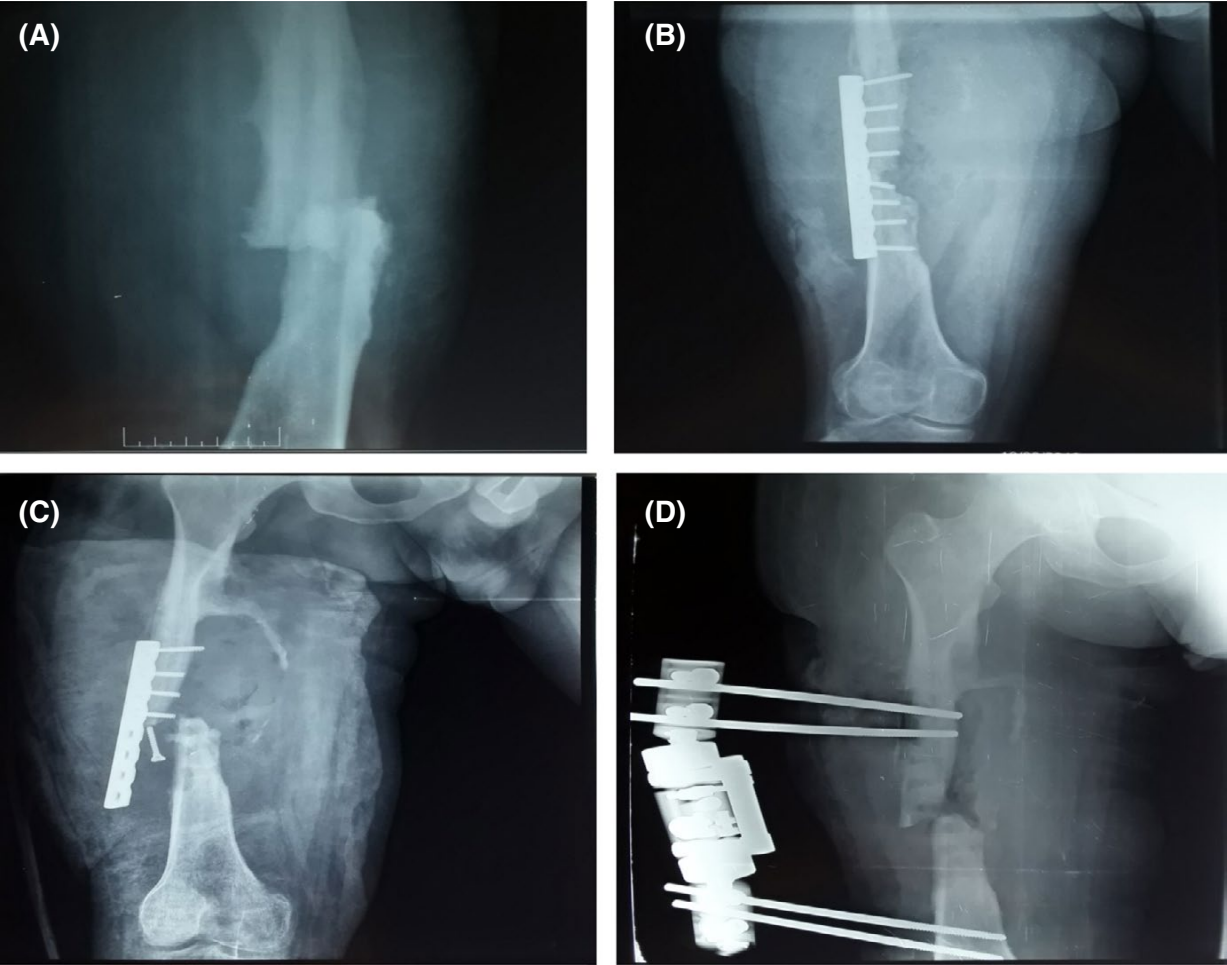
A, Anteroposterior (AP) radiograph demonstrating a comminuted femoral shaft fracture in a 37‐y‐old male patient with hemophilia B. B, Postoperative radiograph of the femur after plate fixation. C, AP radiograph of the femur at postoperative 1 mo demonstrating a nonunion of the femoral shaft and displacement of osteosynthesis material. D, AP radiograph of the femur during a period of short supply in bypassing agents demonstrating a nonunion of the femoral shaft after external fixator
